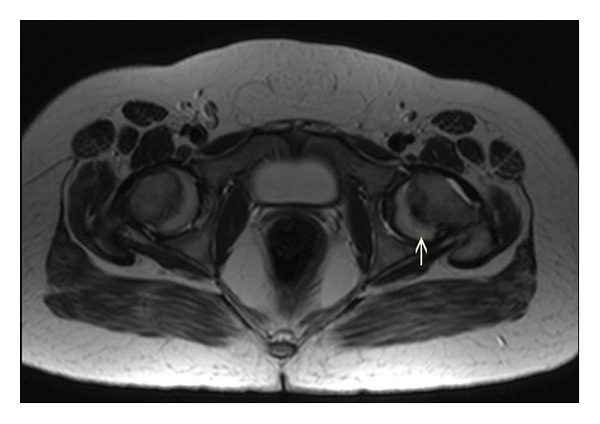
Axial T2-weighted MR image at the level of bilateral hip joint showing posterior displacement of the epiphysis of femoral head on left side (arrow).
